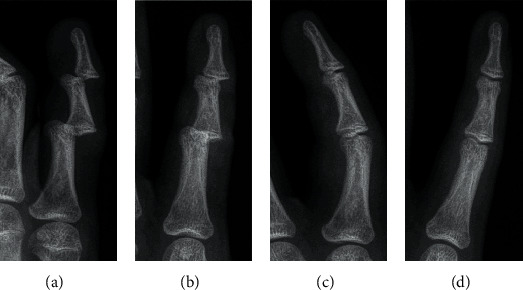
Anteroposterior and lateral radiographs (a, b) of the index finger showing dorsal dislocation of both the proximal and distal interphalangeal joints, without any bony avulsion fracture. Postreduction anteroposterior and lateral radiographs (c, d) confirm that all joints are well reduced.
